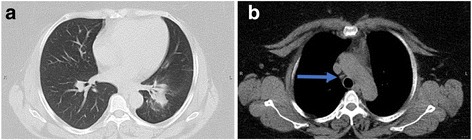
Repeat chest computed tomography revealed a decrease in the size of the lung mass and lymphadenopathy. Following 3 months of high-dose prednisone therapy, repeat computed tomographic scan revealed a significant decrease in (a) the size of the lung mass, which measured 4.7 × 3.1 × 5.8 cm, and (b) lymphadenopathy (*arrow*)
